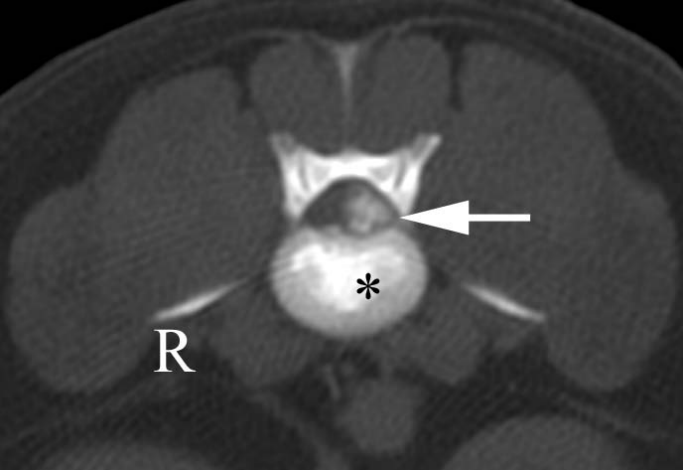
CT scan in bone window through L2-3 showing calcified material (arrow) to the left in the spinal canal. The intervertebral disc is calcified (*). These findings suggest extruded disc material causing a severe lateral compression of the spinal cord that was confirmed at surgery.
